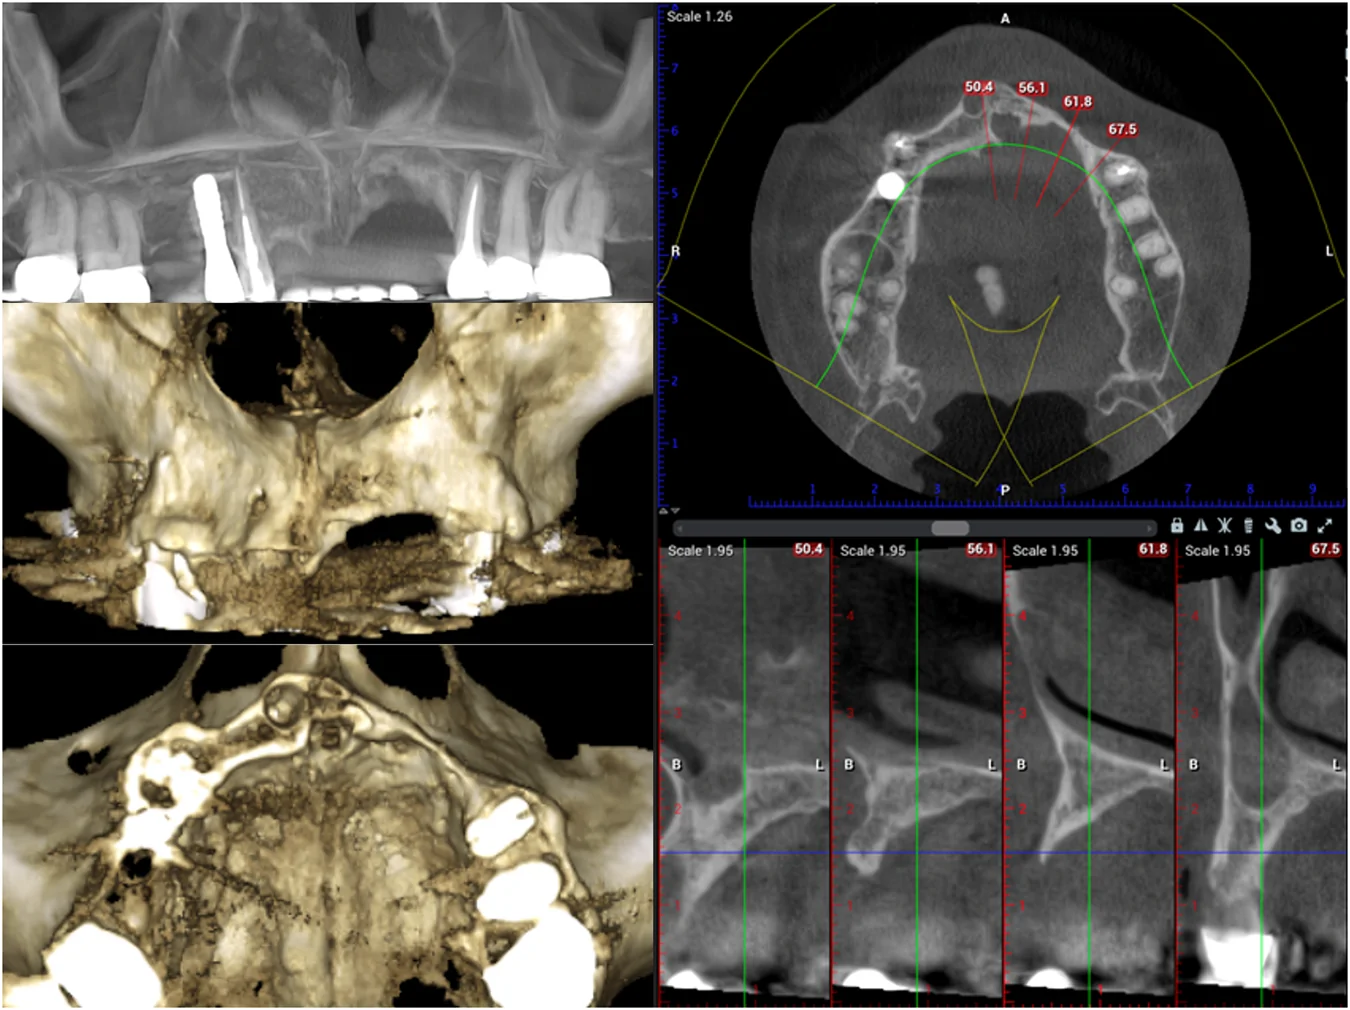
Case 1 Pre-op. Cone beam CT (CBCT) images show an area of extensive loss of bone height on the reconstructed panoramic radiograph and loss of bone width in the axial plane and cross-sectional slices. Top left: Panoramic radiograph. Middle left and bottom left: 3D reconstruction. Top right: CBCT axial view. Bottom right: CBCT coronal view. The numbers in the axial view represent angular positions of the gantry relative to the patient and match the coronal sections.
